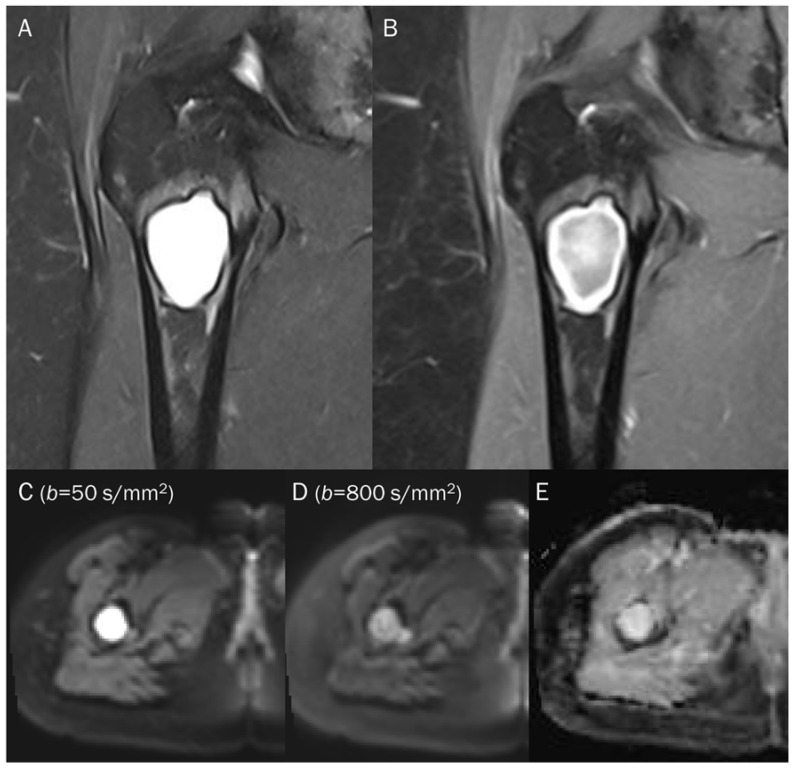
A 38-year-old female with cystic degeneration of fibrous dysplasia in the proximal femur. (A) Coronal T2-weighted fat-suppressed and (B) T1-weighted enhanced images show an intramedullary, lobulated bony mass with a bright signal intensity mass and heterogeneous enhancement in the proximal femur. (C,D) DWI with *b*-values of 50 and 800 s/mm^2^ exhibit slight signal loss of the tumor as the *b*-value increases. However, hyperintensity remains in high *b*-values, raising the possibility of “restricted” diffusivity. (E) ADC map shows little signal reduction in the ADC map, indicating a T2 “shine-through” effect, possibly due to the high T2 relaxation time of the cystic change of the tumor.
